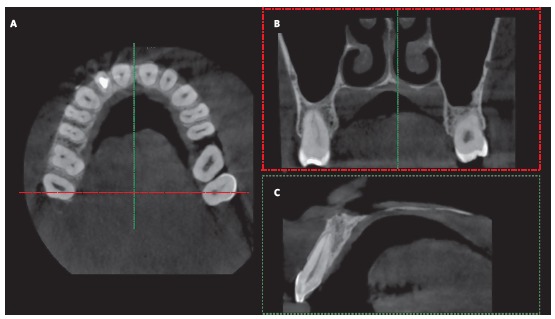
CBCT images. A) Axial section. B) Coronal section at the region of dotted lines (in red, in A). C) Sagittal section at the level of dotted lines (in green, in A), evidencing scarce bone tissue for anchorage of miniscrews in MARPE.
